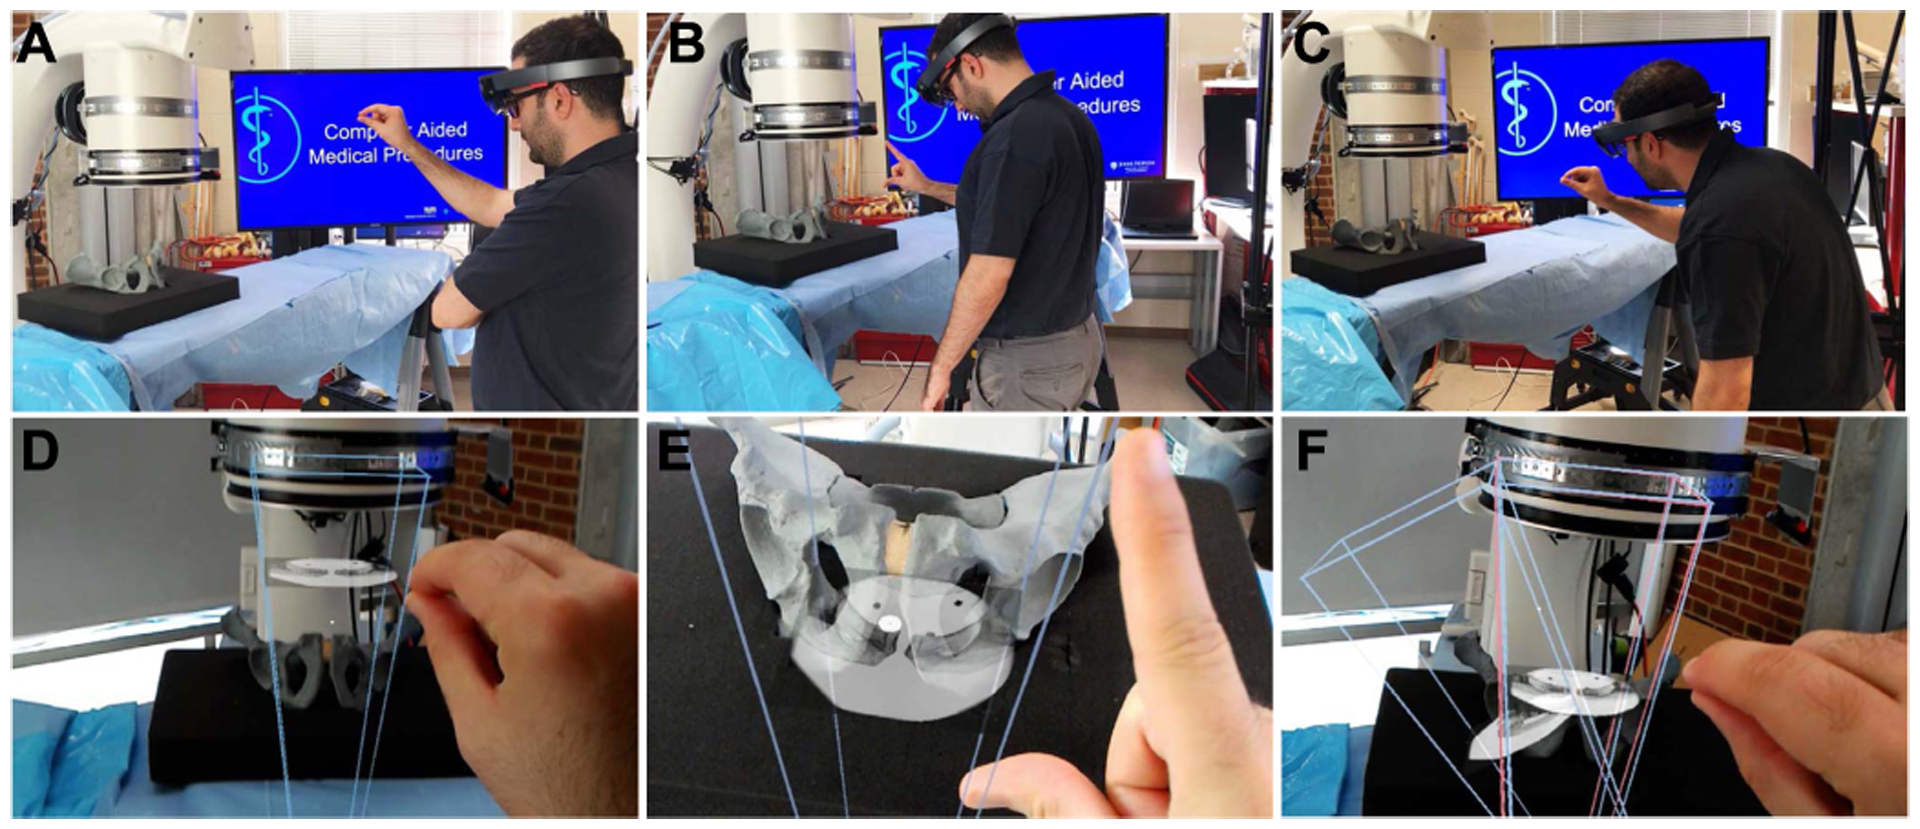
Spatiotemporal-aware AR exploits the full imaging geometry. The augmented user interacts with the X-ray images within their viewing frustums (A-C). Corresponding AR views are shown in D-F.
